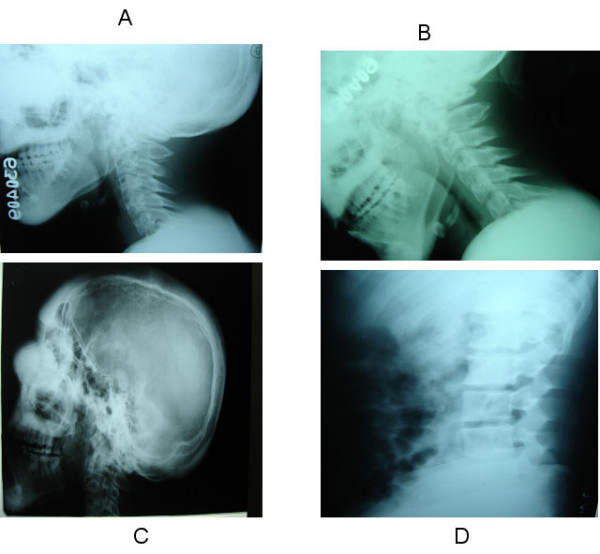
Panel A and B show the cervical spine Xray in neutral position and flexion respectively. Note the hypertrophy of the posterior elements. Panel C shows diffuse cortical thickening on a lateral skull X-ray with soft tissue thickening and elevation of the nasal bridge. Panel D shows diffuse thickening of the posterior elements of the lumbar vertebrae.
